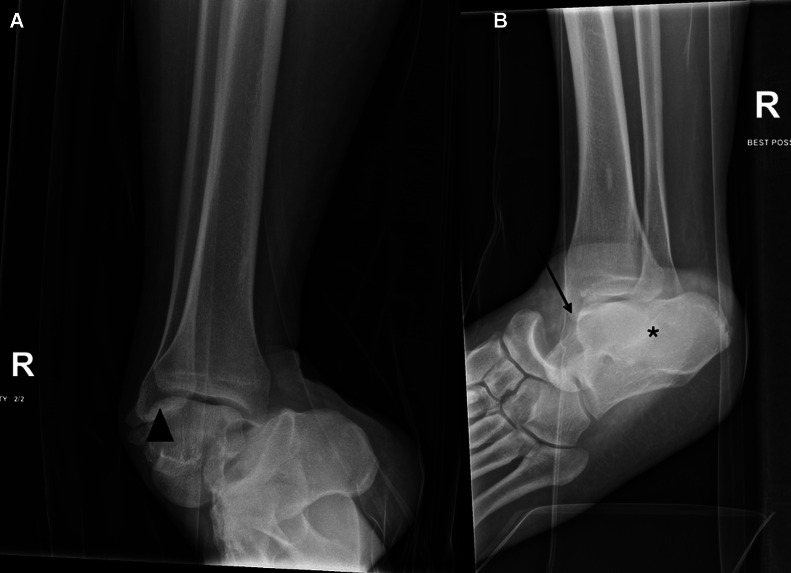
Best possible anteroposterior (A) and lateral (B) radiographs of the right ankle. There is tri-articular dislocation of the talonavicular (arrow), subtalar (asterisk), and tibiotalar (arrowhead) joints.
